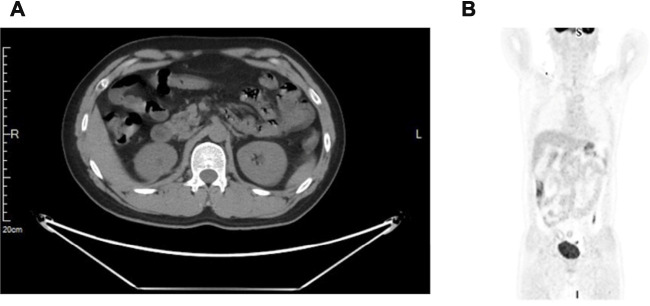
A positron emission tomography-computed tomography (PET/CT) scan. (A) No suspicious primary lesion recurrence in the right kidney after surgery. (B) Neither lymph nodes enlargement adjacent to the aorta nor remote organ metastases.
